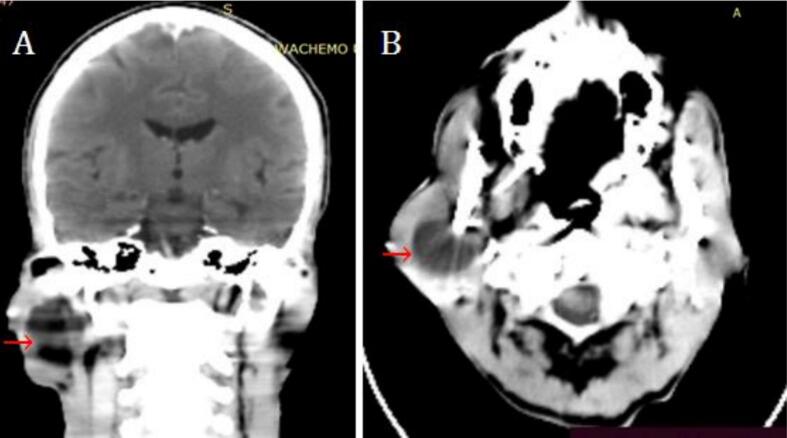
The CT scan shows cystic right parotid lesion (red arrows). (For interpretation of the references to colour in this figure legend, the reader is referred to the web version of this article.)
